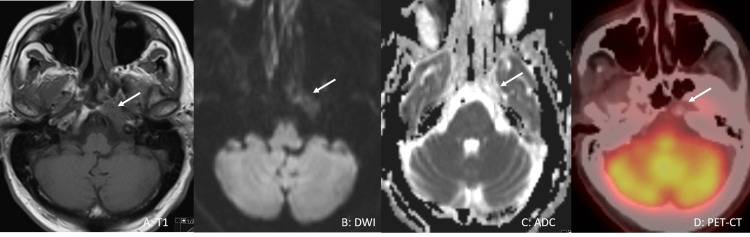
Follow-up MRI Follow-up MRI demonstrates a reduction in the size of the lesion in the left clivus (arrow in A) as well as resolution of diffusion restriction. The lesion now demonstrates a high signal on both diffusion-weighted image (DWI) and apparent diffusion coefficient (ADC) images (arrow in B and C) consistent with facilitated diffusion. Positron emission tomography CT (PET-CT) (D) shows no evidence of fluorodeoxyglucose (FDG) uptake within the lytic lesion (arrow).
